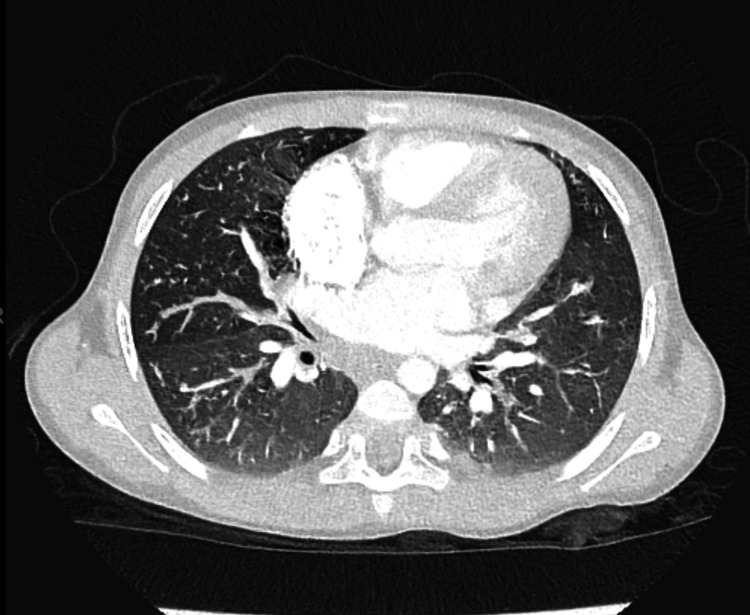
Chest CT showing mucus impaction
